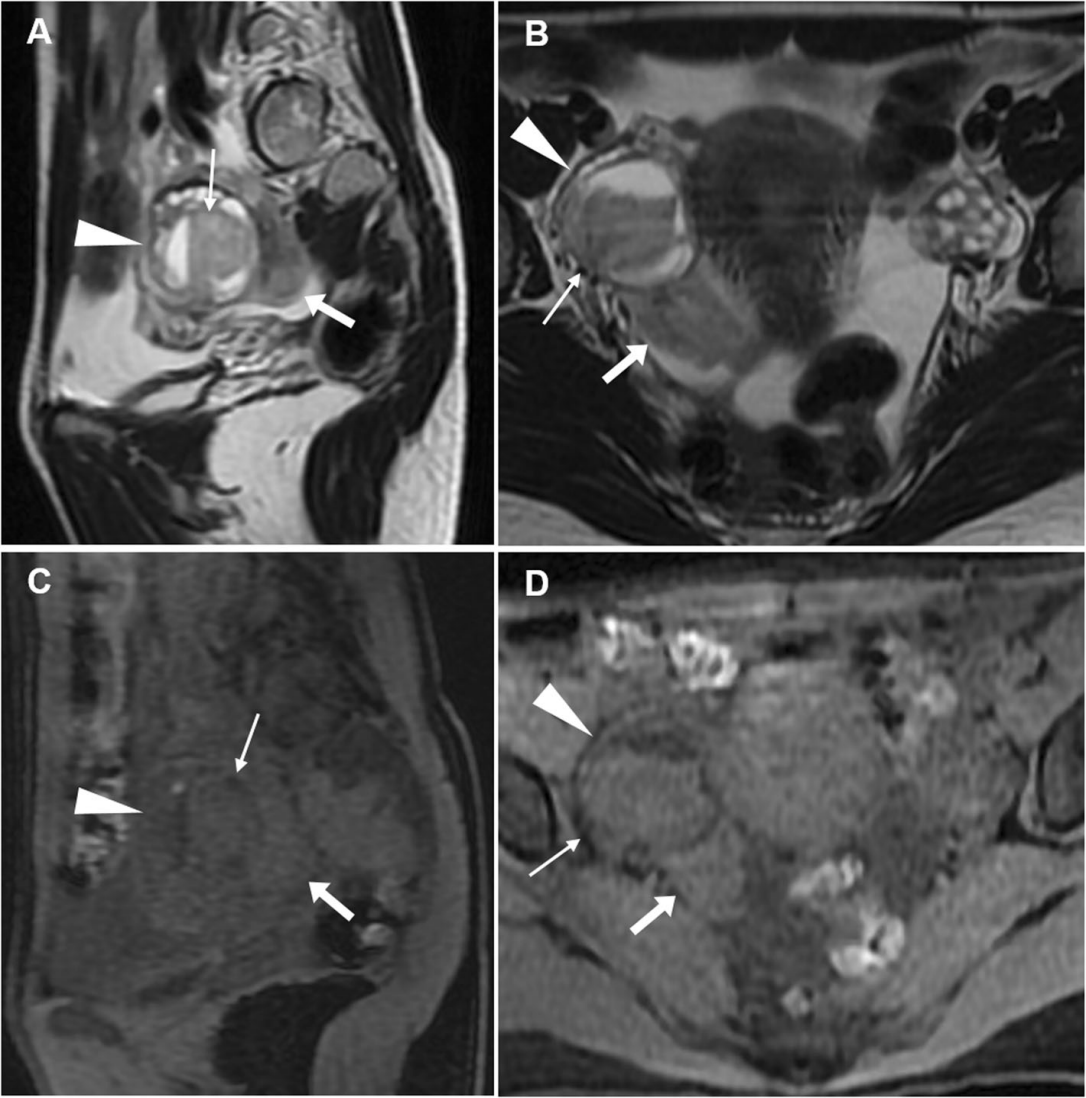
Ruptured haemorrhagic corpus luteum with haemoperitoneum in an 18-year-old woman with pelvic pain for 24 h. Sagittal (a) and oblique-coronal (b) T2-weighted, sagittal (c) and oblique-coronal (d) precontrast fat-suppressed T1-weighted images show right-sided ovarian cyst (arrowheads) with internal fluid-fluid level and a dependent component with intermediate signal intensity on T1- and T2-weighted images (thin arrows), corresponding to recent haemorrhage (< 48 h). Note the haemorrhagic peritoneal effusion with intermediate signal intensity on both T1 and T2-weighted images adjacent to the posterior aspect of the cyst (arrows)
